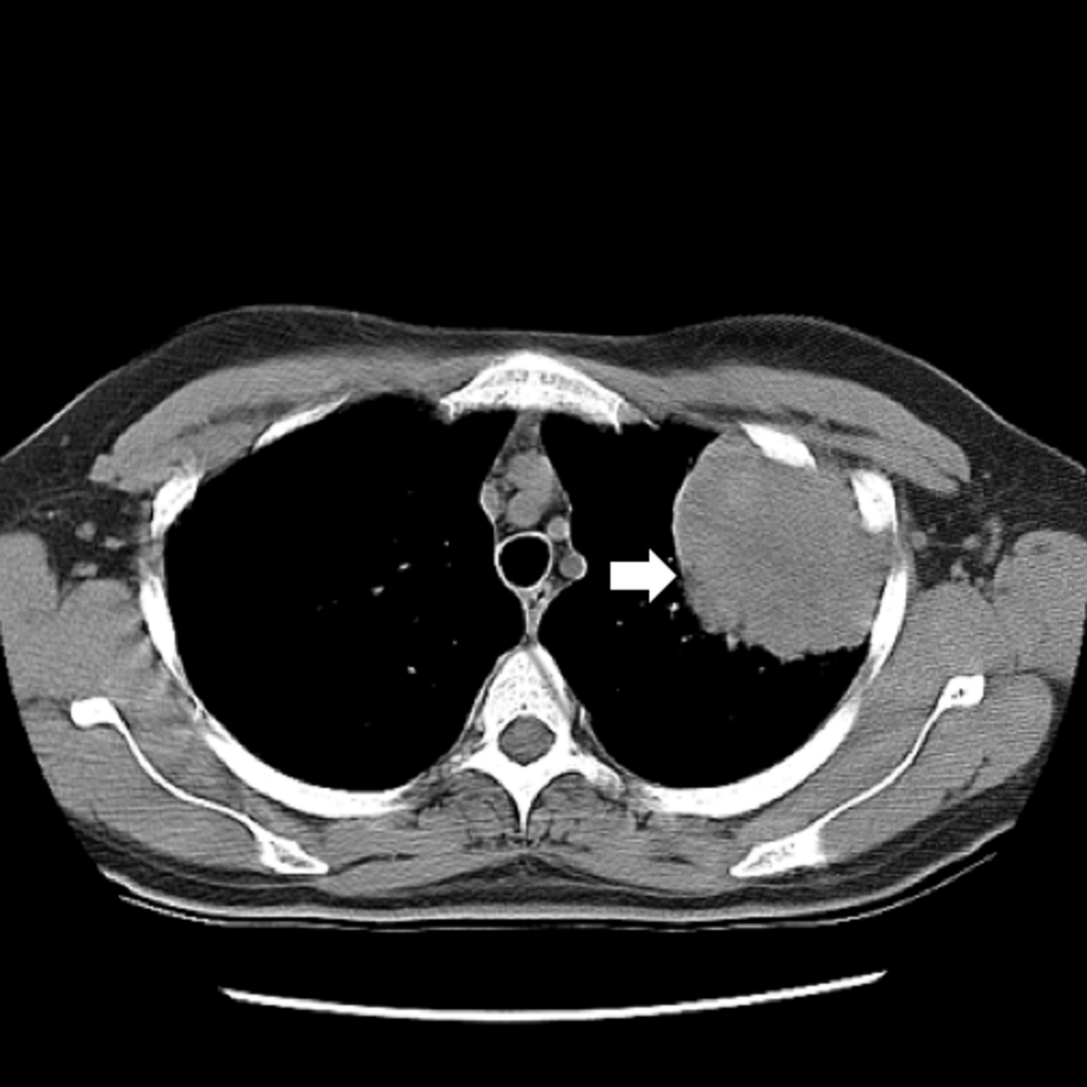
Case 1: chest imaging Computed tomography of the chest at presentation showing a 7 x 5-cm left upper lobe mass.
